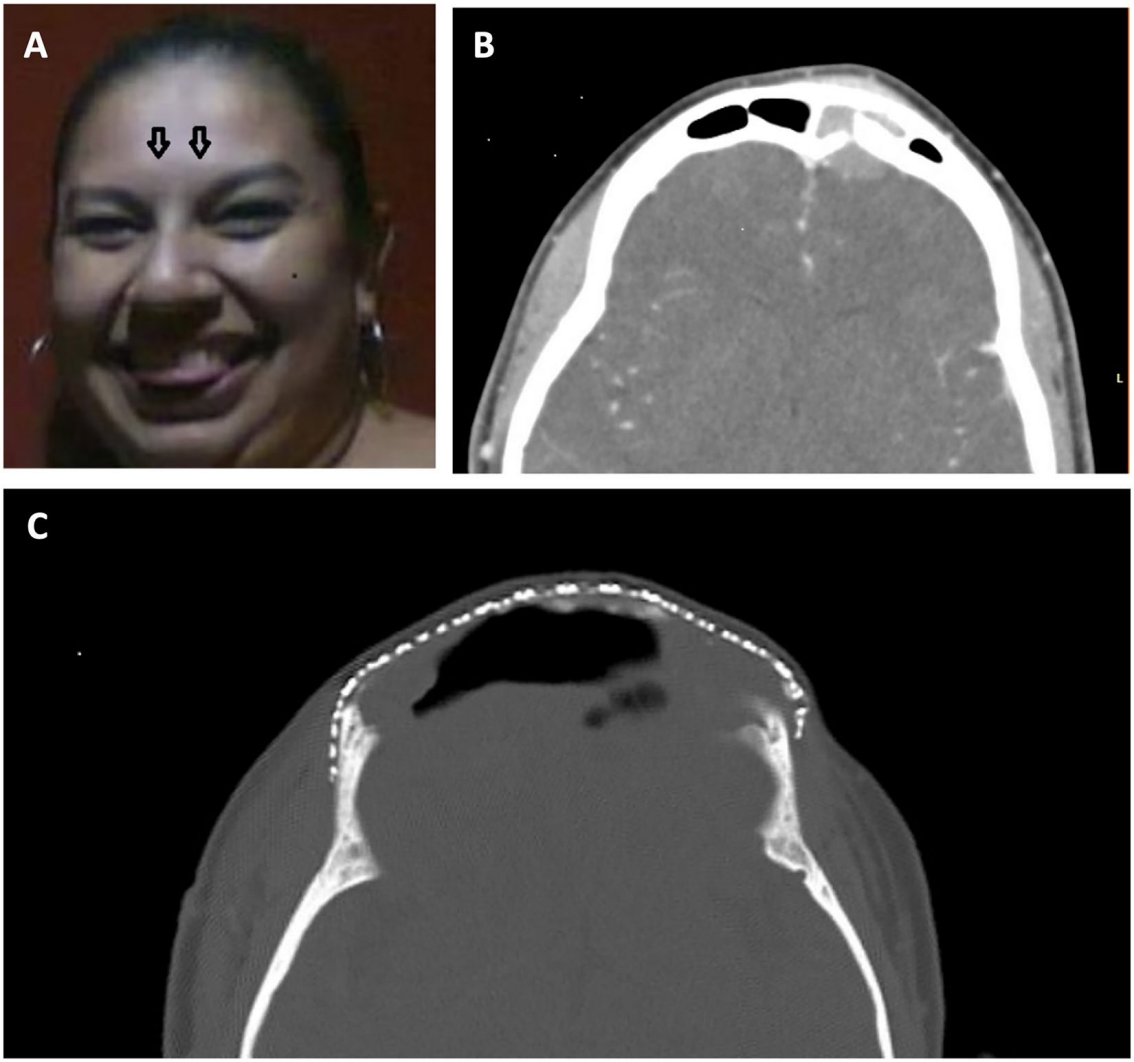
(A) Painful forehead lump (noted in the spring of 2013). (B) Computed tomography demonstrates a left frontal area mass extending through the sinus to the frontal lobe. (C) Status post multiple craniotomies and placement of the titanium mesh (2017).
